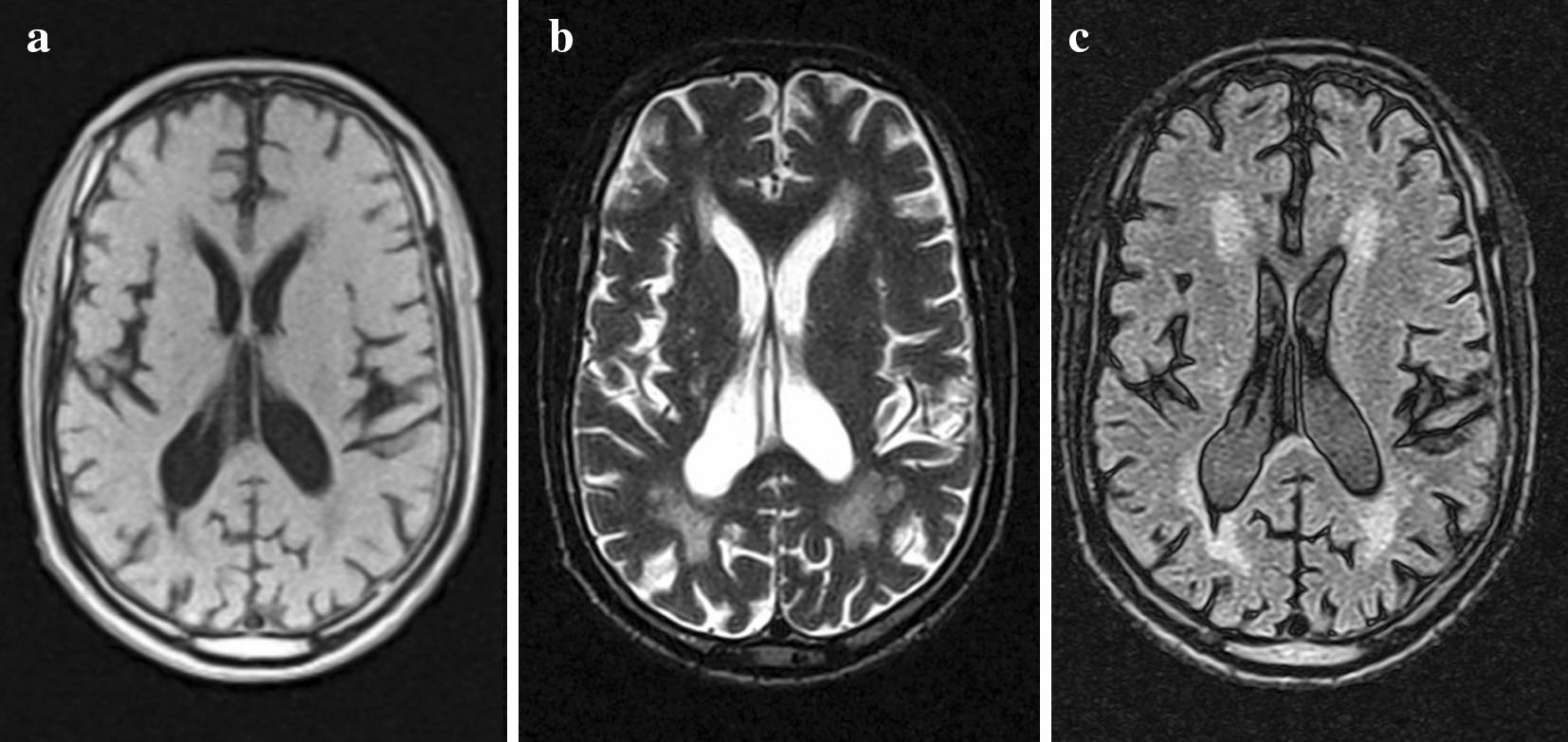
Magnetic resonance imaging (MRI) T1- and T2-weighted (a, b), and fluid-attenuated inversion recovery (c) axial images from a 69-year old male Nigerian stroke survivor showing moderate white matter hyperintensities
